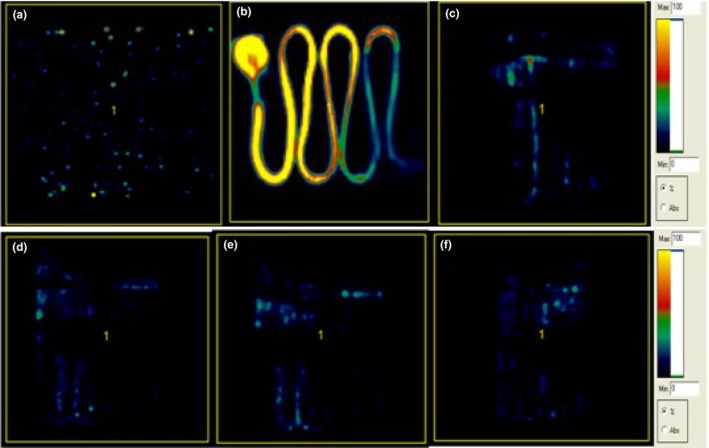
Representative FDG PET images for CR Bard PowerPort^®^ isp (Model 1708560): (a) Saline only, (b) Positive FDG control, (c) After 10 ml of saline flush, (d) After 30 ml of saline flush, (e) After 60 ml of saline flush, and (f) After 120 ml of saline flush.
